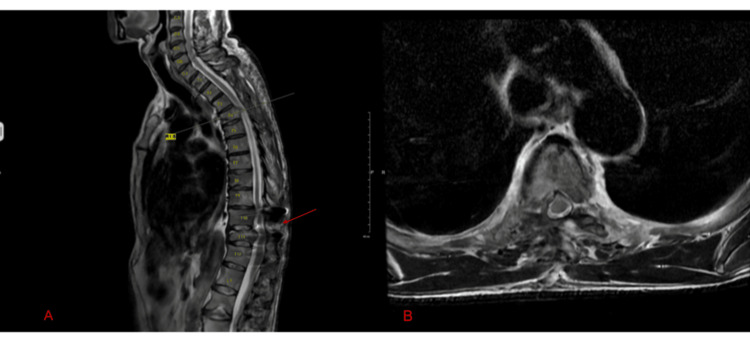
A: Thoracic MRI saggital view demonstrating adequate decompression with minor signal changes at T10-T11 levels (red arrow); B: Axial view through T4 demonstrating adequate decompression
